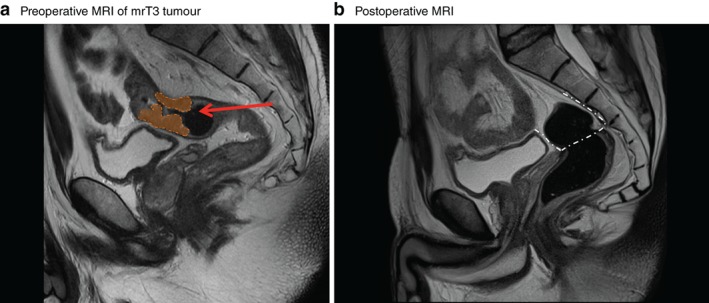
Sagittal T2‐weighted MRI of the pelvis
